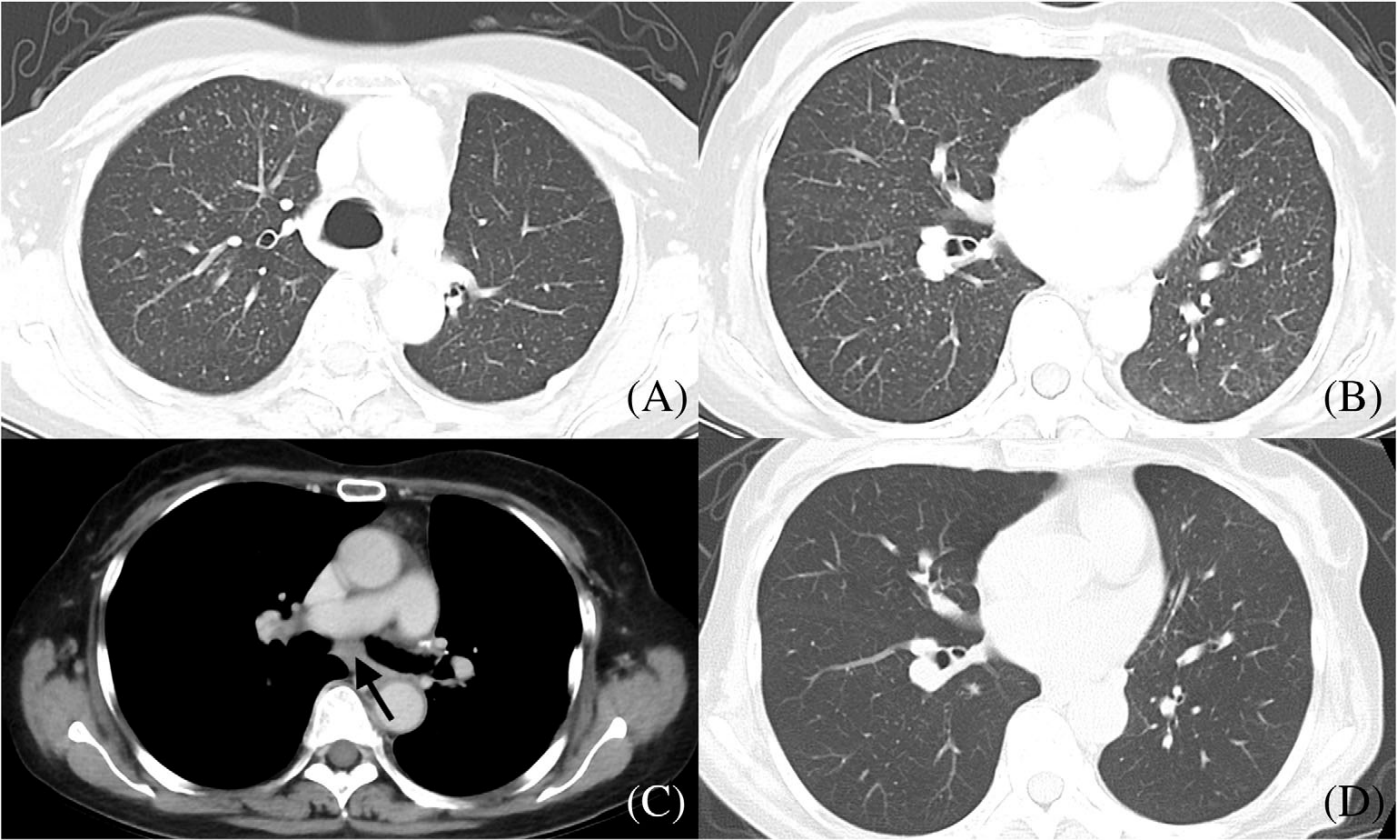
Thoracic computed tomography (CT) scan finding of the patient with miliary nodules. (a, b) CT showed the presence of miliary nodules. We performed transbronchial lung cryobiopsy (TBLC) and transbronchial lung biopsy with forceps (TBLB) to these areas and TBLC detected malignant tumors. (c) CT revealed swollen mediastinal lymph nodes. Transbronchial needle aspiration (TBNA) of the lymph node was performed but malignant cells were not detected. (d) CT did not reveal any miliary nodules after treatment with osimertinib
